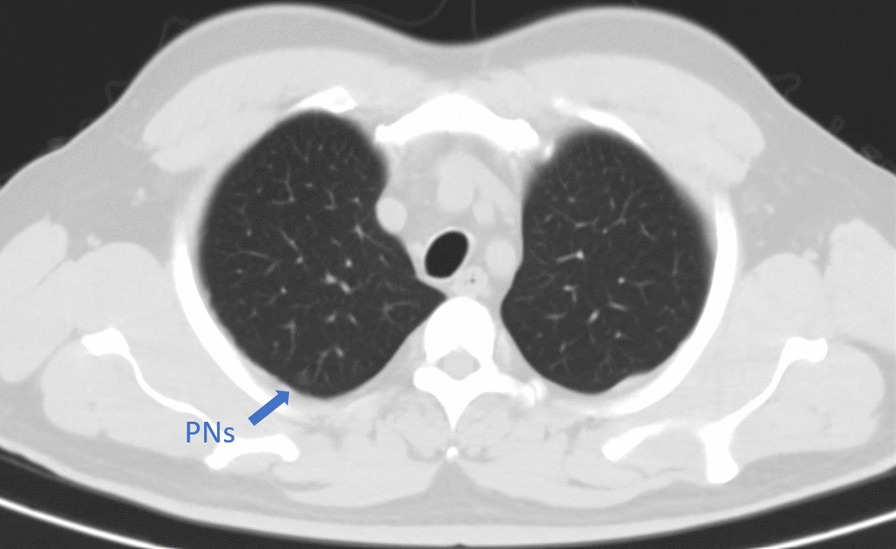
CT scan images of the targeted nodules
